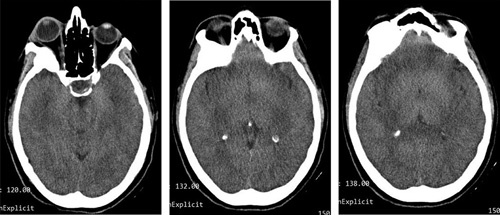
The head computed tomography scan revealed a hyperdense lesion arising from the right greater wing of the sphenoid bone and compressed on the orbit’s components and eye muscles, which caused proptosis.
